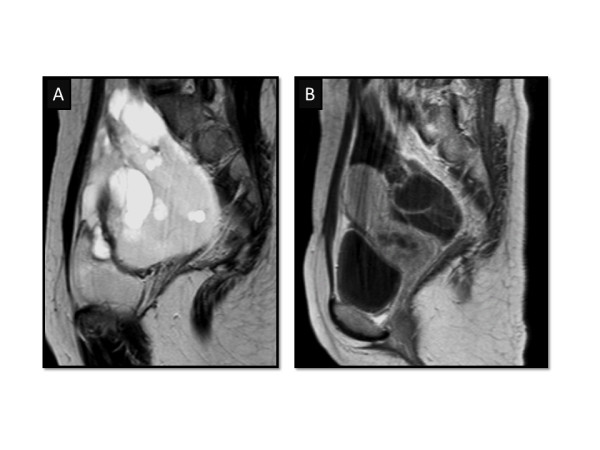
Magnetic resonance imaging of the pelvis on a (A) T2-weighted and (B) gadolinium enhanced T1-weighted image. The cyst walls were partly enhanced with gadolinium administration.
